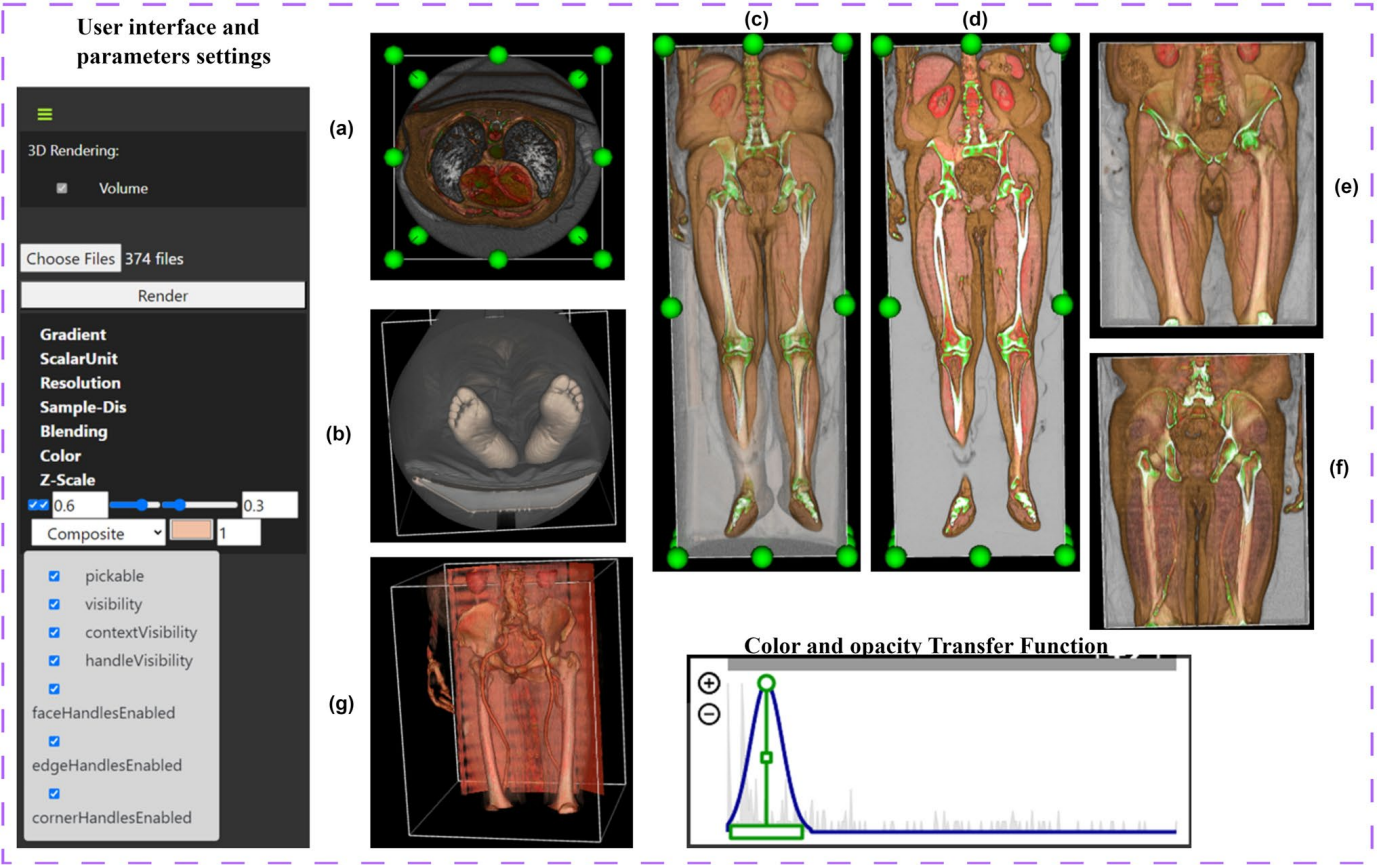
Visualization and Parameter Effects on Peripheral Artery CT Images of Patient #17: (a) Top Series Axial View, (b) Bottom Series Axial View, (c) Coronal View, (d) Cropped Coronal View, (e) Cropped Front Coronal View of Region of Interest, (f) Cropped Back Coronal View of Region of Interest, (g) Enhanced Detailed View of Pelvic Arteries with Transfer Function Adjustments
